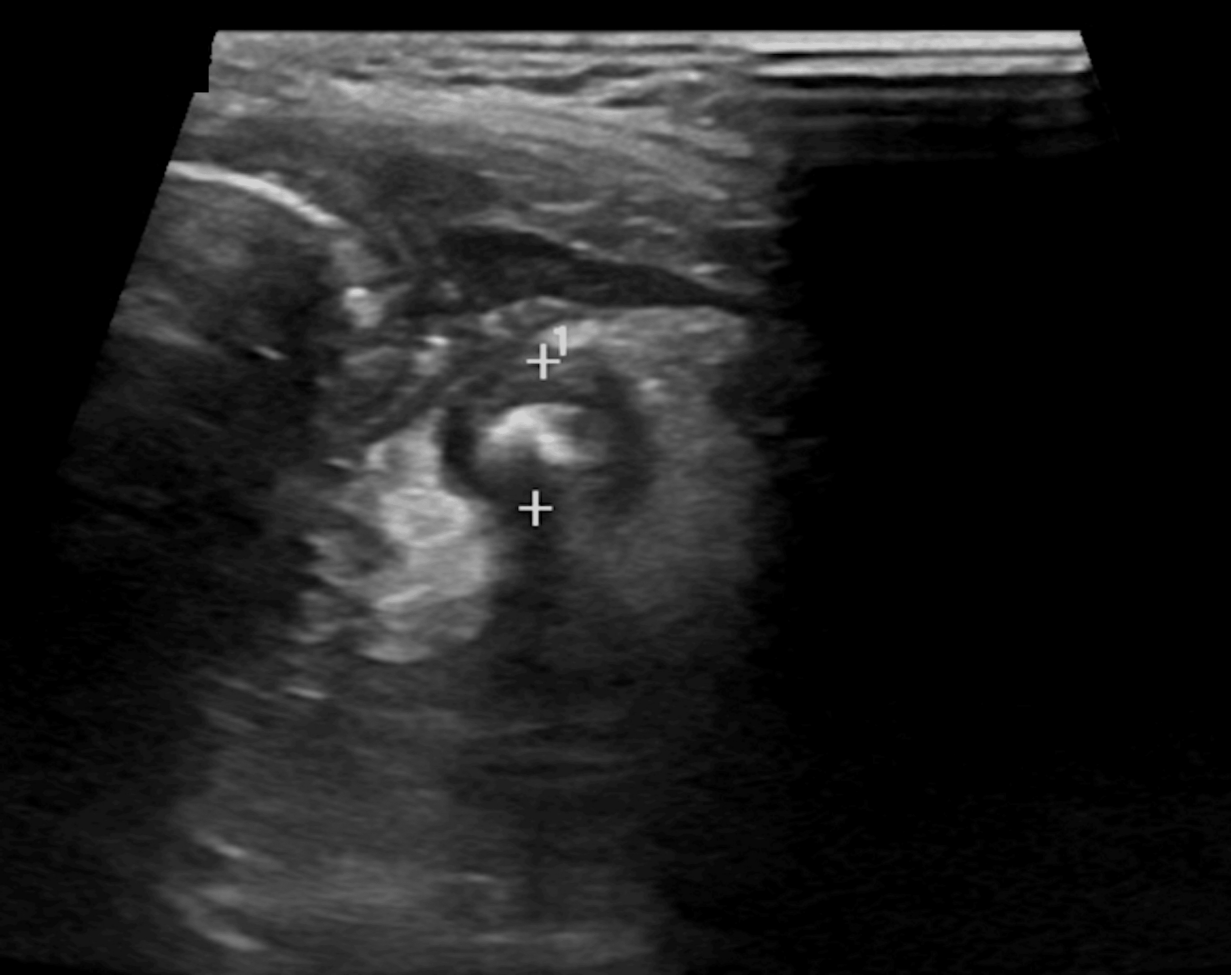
Abdominal ultrasound of the right lower quadrant demonstrating calcifications likely representing an appendicolith.
